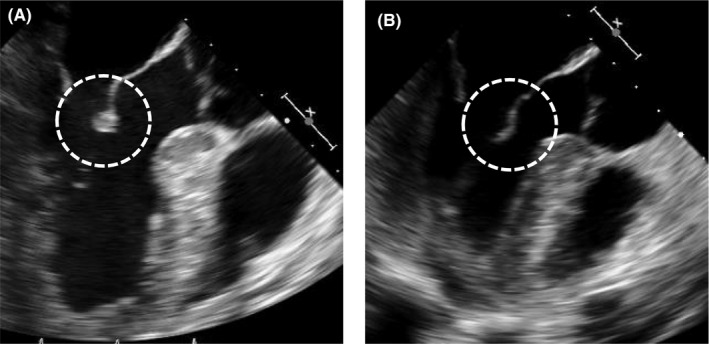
The pictures show mitral valve lesion on transesophageal echocardiography (circle). A, shows before treatment, and (B) shows after treatment
